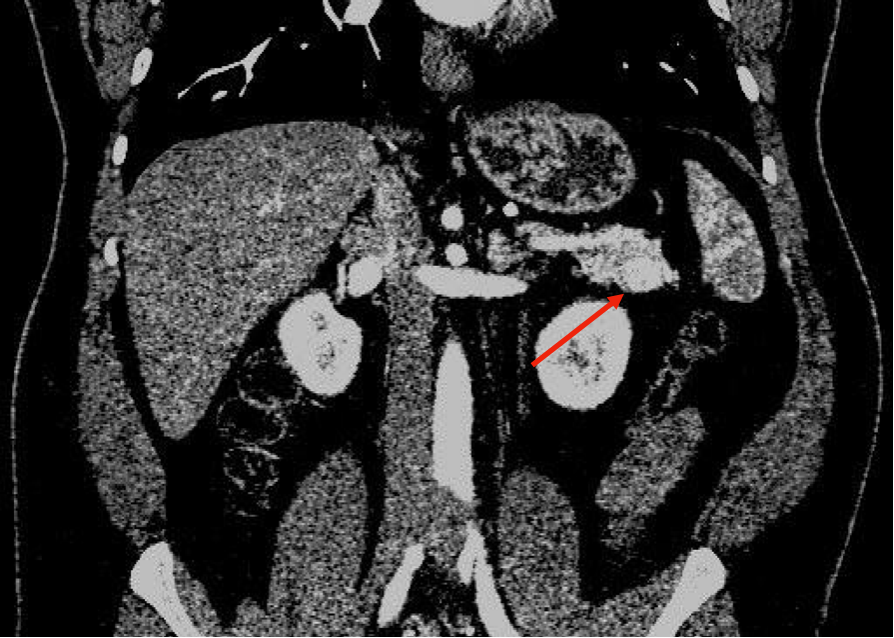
Triple phased abdominal computed tomography scan: arterial phase, coronal view. 1.8 × 1.6 hypervascular mass at the tail of pancreas (arrow)
